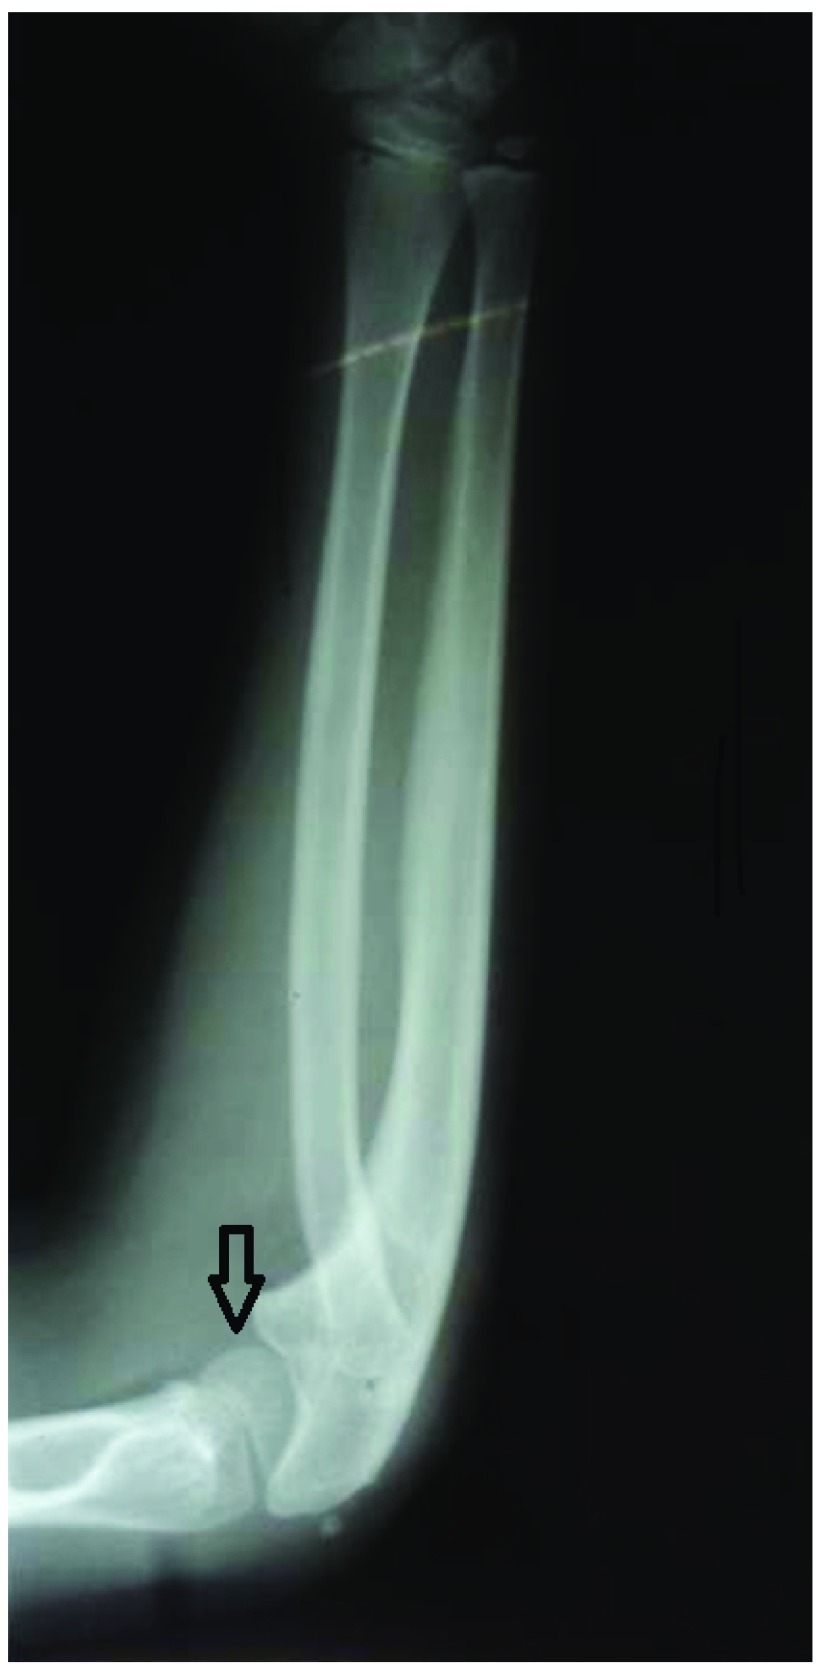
Pre-operative X-ray showing congenital radial head dislocation with the radius bowed anteriorly. The capitulum is hypoplastic.
